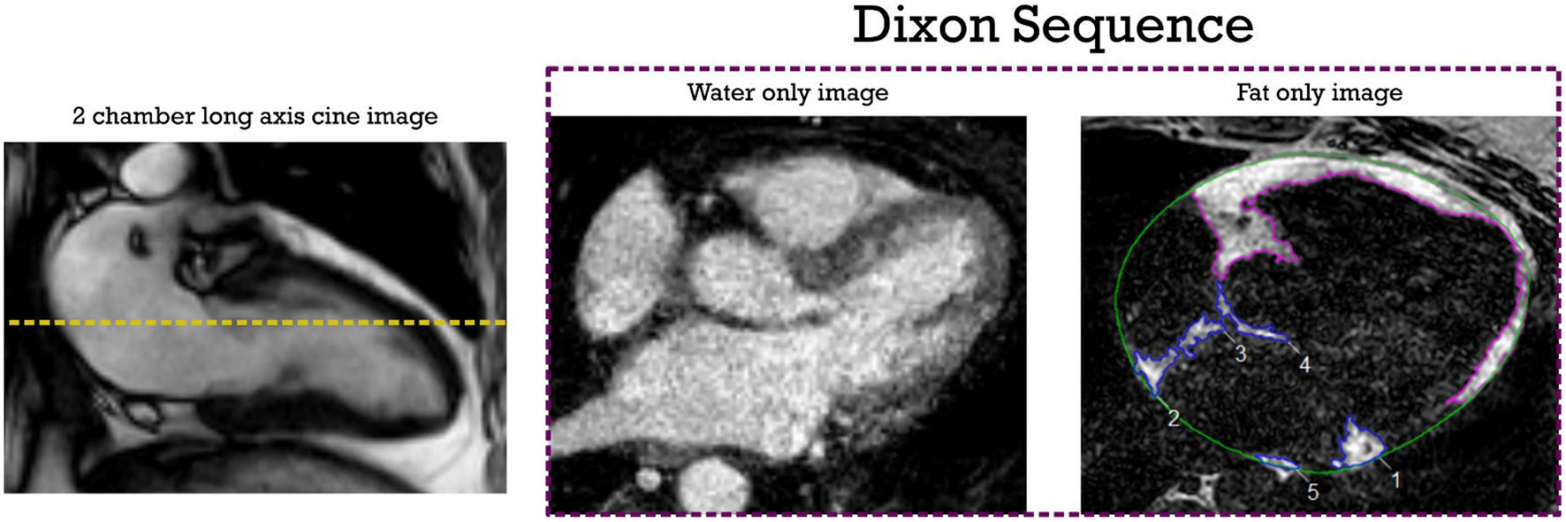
EAT segmentation from axial Dixon MRI sequence. The dotted yellow line shows the measurement level in the LA. High intensity signal areas around LA (inferior pulmonary vein level in this example) are manually contoured in blue using point by point contour tools. Ventricular EAT is contoured in purple; Total epicardial fat is calculated by adding atrial and ventricular EAT across all slices. The pericardial outline is shown in green. EAT, epicardial adipose tissue; LA, left atrial.
